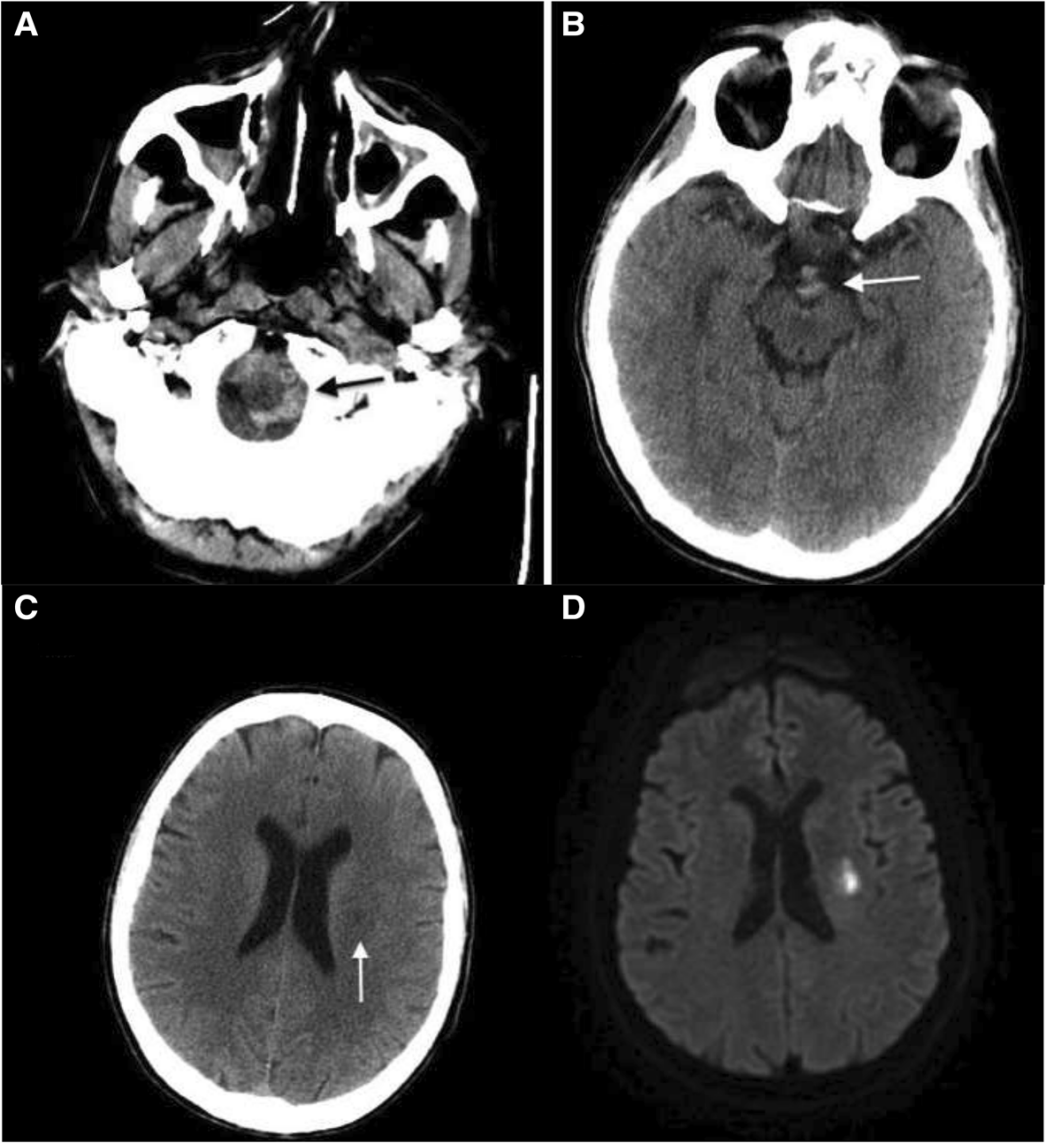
Initial non-contrast head computed tomography scan shows subarachnoid hemorrhage (arrows) (A, B); subsequent scan (C) shows ischemic lesion (arrow) that diffusion-weighted magnetic resonance imaging (D) confirms to be consistent with acute infarct.
